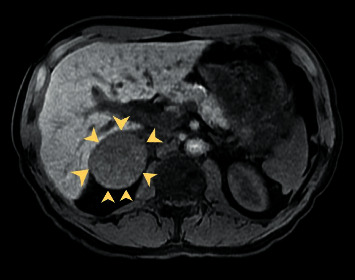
T2-Weighted MRI of the abdomen: slight increased T2 signal and heterogeneous enhancement of the right adrenal gland (arrows).
